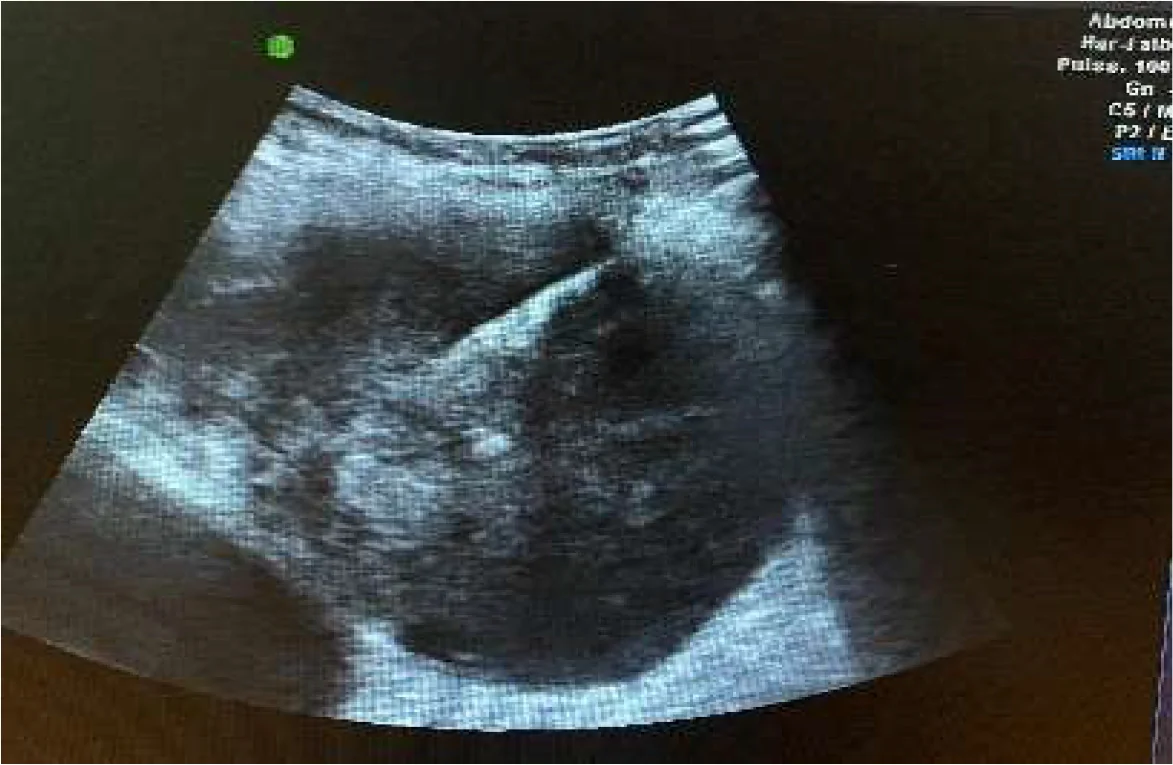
Ultrasound-guided biopsy of the solid component of the tumor using a 14-Gauge semi-automatic needle.
